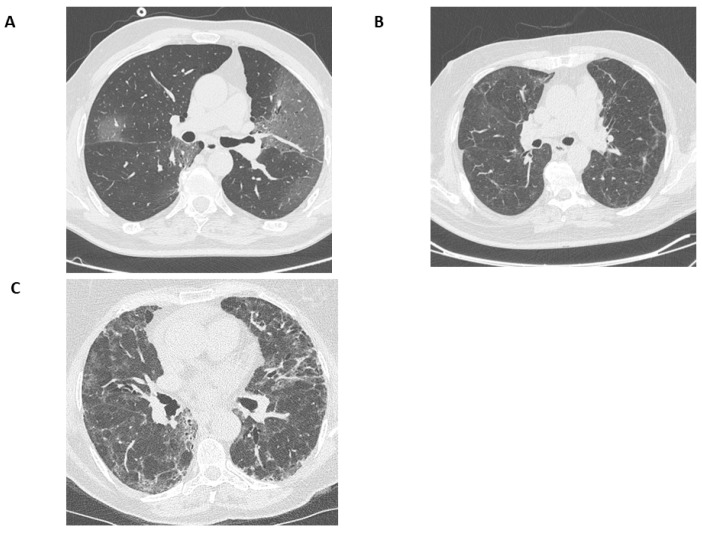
The radiological patterns assessed during the evaluation of follow-up HRCT after hospital discharge: (A) COVID-19-associated pulmonary opacities, (B) parenchymal bands, and (C) fibrotic-like changes. HRCT = high resolution computed tomography.
